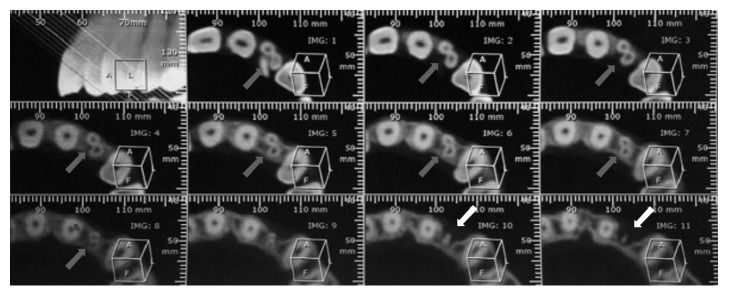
3D reconstruction of CBCT images with 0.25 mm axial slice thickness and 0.25 mm slice interval. Images 1–8 indicate the presence of two root canals in the maxillary left lateral incisor. Images 10 and 11 show apical rarefaction with destruction of the vestibular cortical bone.
